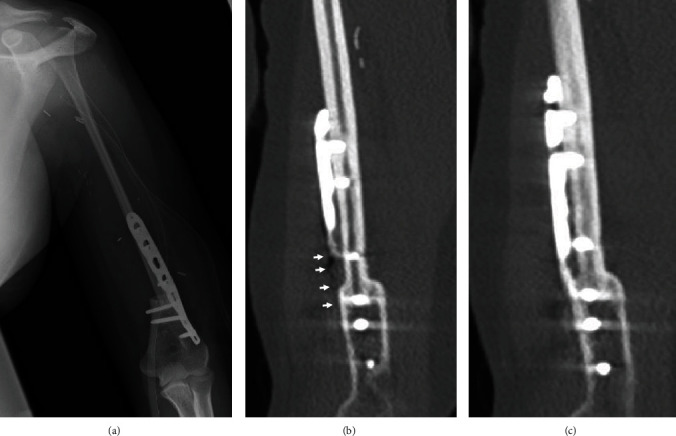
Postoperative findings of radiographs and computed tomography. (a) Postoperative radiographs showing the distal end of the free vascularized fibular graft fixed to the proximal stump of the humerus with a plate; sling procedure. (b) Postoperative CT scan showing the distal end of the grafted bone was fixed to the proximal stump of the humerus with a locking plate and suspended the head of the fibula for reconstruction of the shoulder joint with the tendons of the biceps femoris and palmaris longus. (c) Computed tomography taken five years postoperatively showing the formation of callus between the graft bone and humerus.
